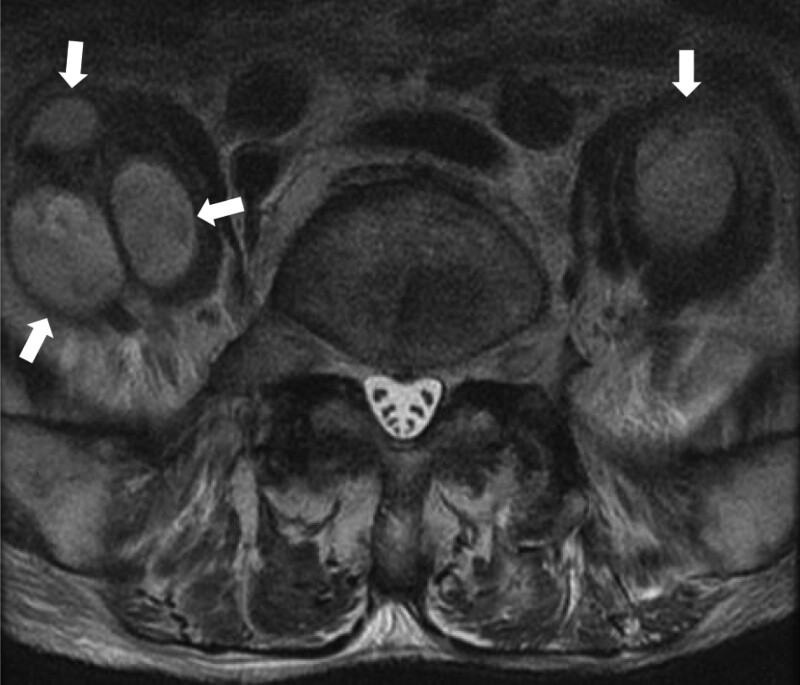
The magnetic resonance imaging (MRI), coronal T2-weighted image in case 1 of series 1. The arrows show in this MRI show high intensity-imaging of multiple abscesses in bilateral psoas muscles surrounded by low intensity capsules.
